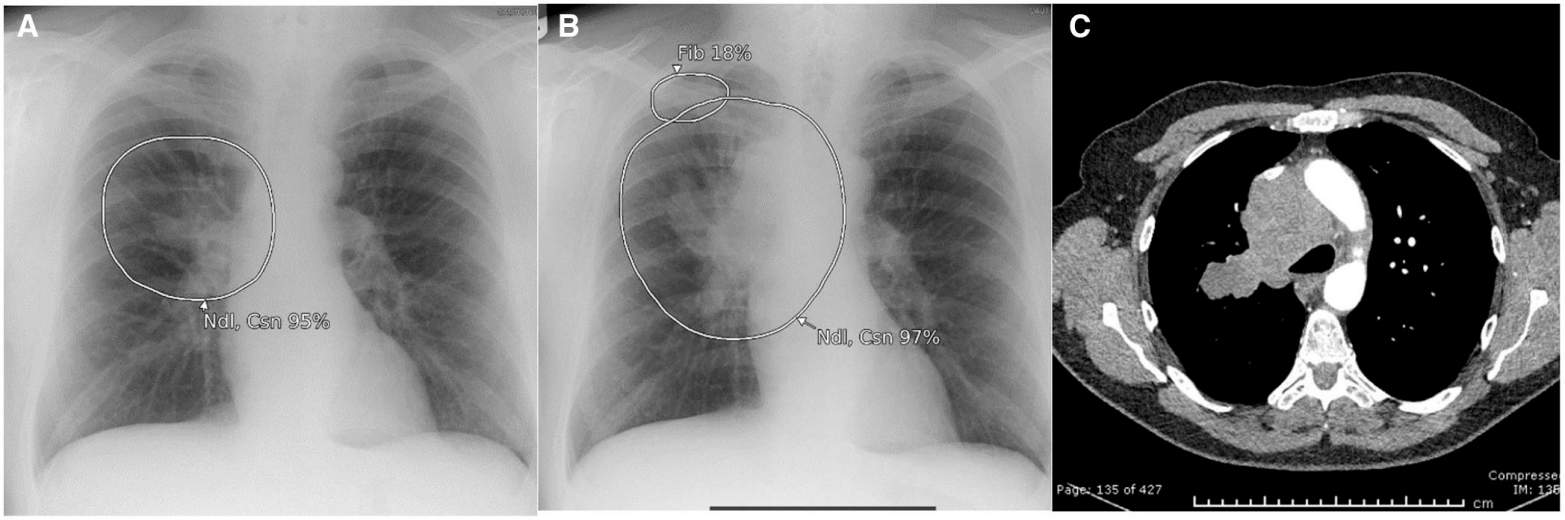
A 69-year-old male smoker with a history of haemoptysis and a cough underwent a CXR (A), report as NAD and *normal hilar shadows*. The AI software highlights a large suspicious nodule in the right hilar region. Eleven months later, the patient has another CXR (B), due to haemoptysis and weight loss. Both the report and AI software highlights suspicion of mass in the right hilar region. The patient has a subsequent CT scan (C) demonstrating a right hilar mass confirmed as stage 3b LC. AI = artificial intelligence; CXR = chest radiograph; LC = lung cancer; NAD = no abnormility detected.
